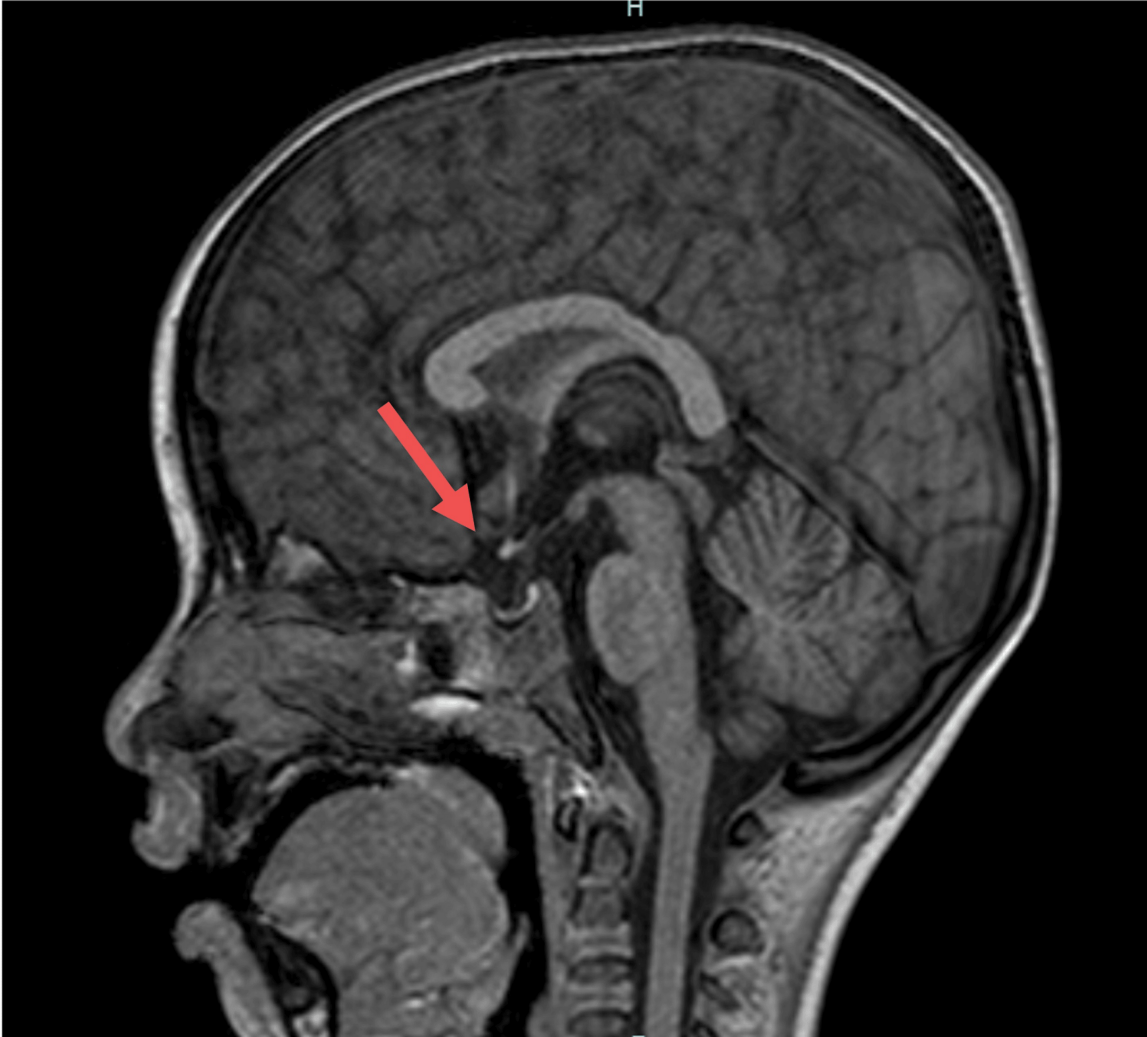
Pre-therapeutic magnetic resonance imaging of the brain Magnetic resonance imaging of the head showed empty sella. There were no findings of optic neuritis, transverse myelitis, acute disseminated encephalomyelitis, or cerebral cortical encephalitis.
